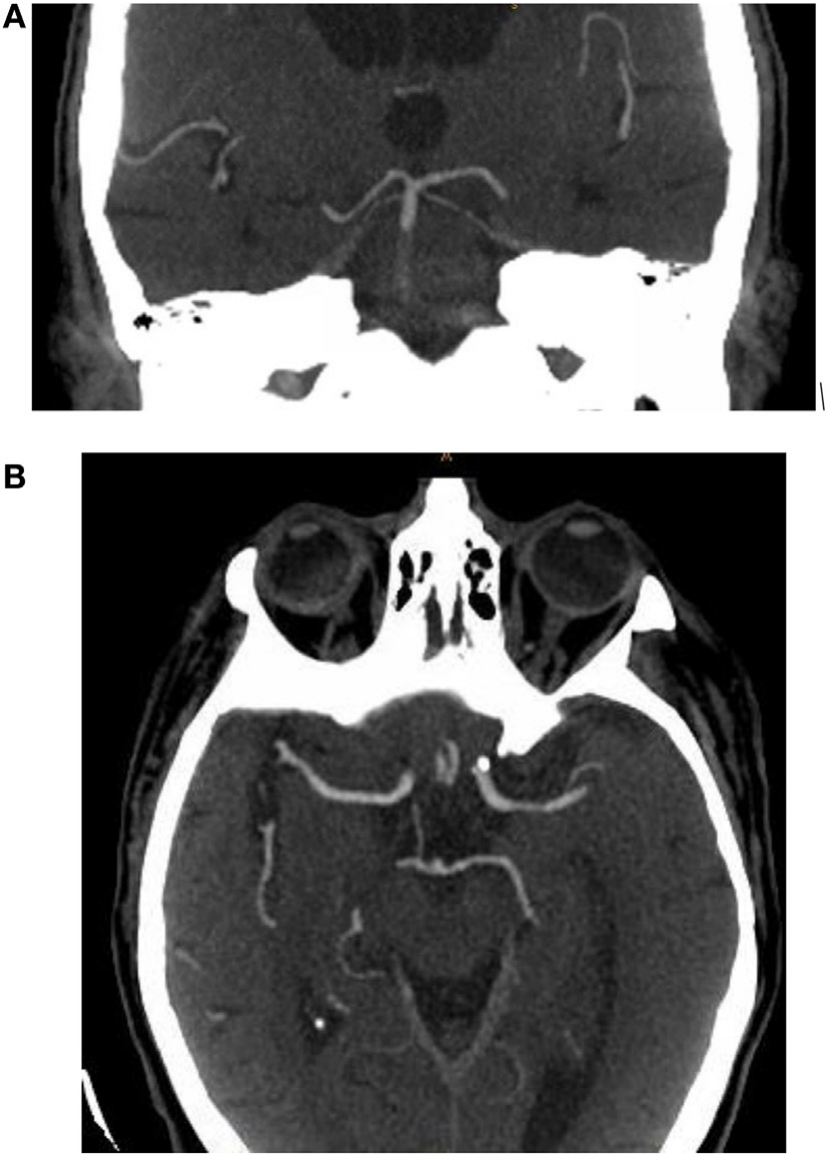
Example of basilar artery on computed tomography angiography (BATMAN) score calculation. Coronal maximum intensity projection of a computed tomography angiogram in a patient with acute basilar artery occlusion. (A) 1 point is assigned to normal arterial filling of the distal segment of the basilar artery and 2 points for normal arterial filling of bilateral P-1 segments of the posterior cerebral artery. (B) Axial maximum intensity projection of a computed tomography angiogram in the same patient. 2 points are assigned to normal arterial filling in the right posterior communicating artery. Total BATMAN score is 5.
